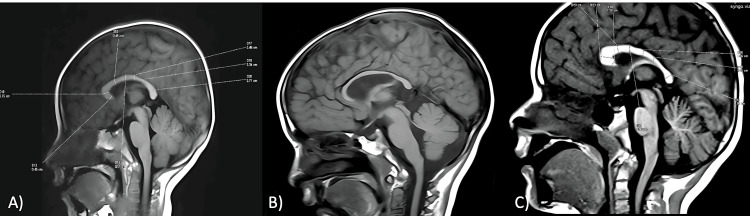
A) Measurement of various parts of the CC on sagittal MRI using Witelson’s method; B) Hypoplastic CC in a child with DD; C) Measurement of the CC in a child with DD CC, corpus callosum; MRI, magnetic resonance imaging; DD, developmental delay
